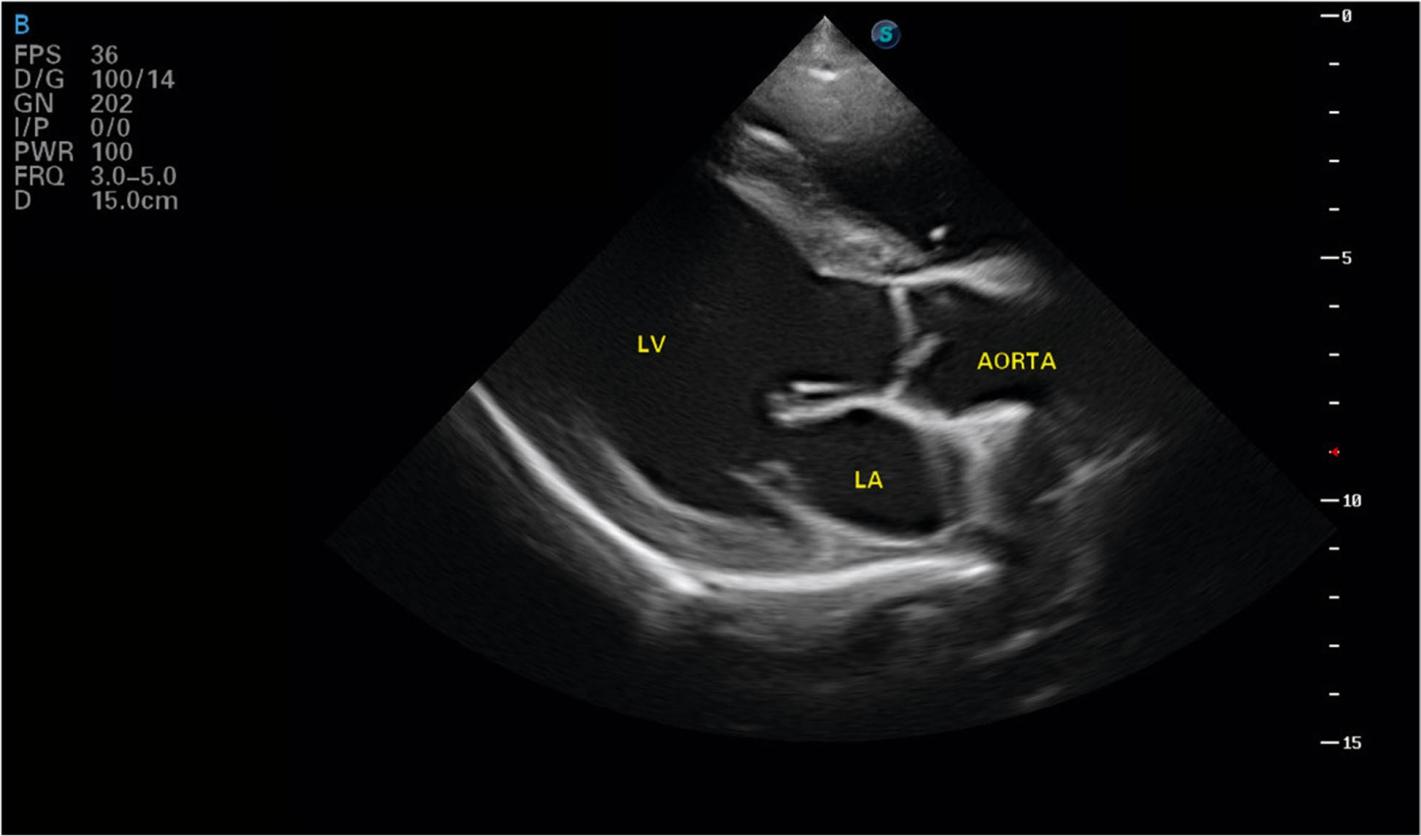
Echocardiography, following resolution of the vegetative aortic lesion (LA: left atrium, LV: left ventricle)
